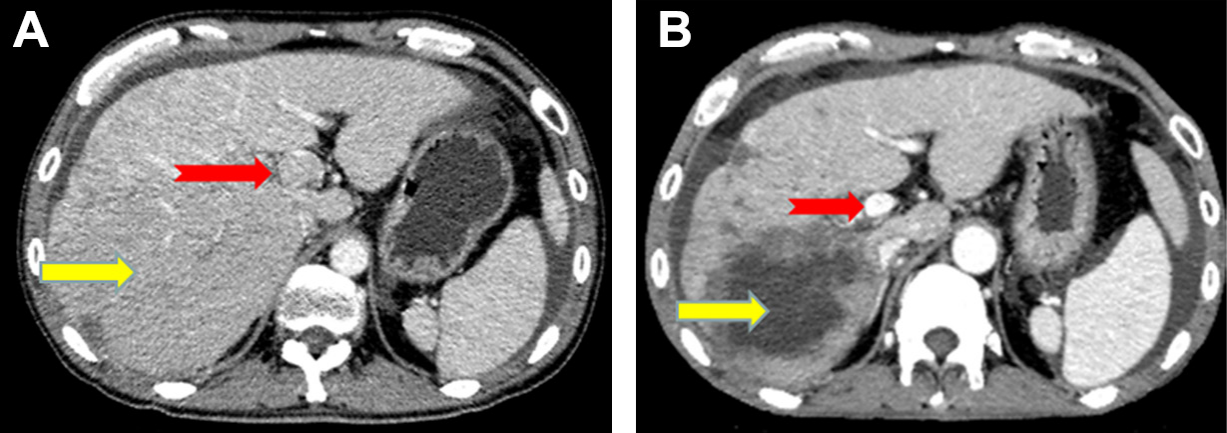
Image of a 48-year-old man with hepatocellular carcinoma and PVTT who showed a partial response after combined apatinib and TACE treatment Contrast-enhanced CT at diagnosis showed a 134 mm diameter hepatocellular carcinoma nodule (yellow arrow) and multiple small metastatic lesions located in the liver, together with PVTT in the left and main portal vein (red arrow). CT images 1 month after diagnosis showed intrahepatic lesions in numerous non-enhanced areas (yellow arrow) and almost complete absence of PVTT without definite enhancement (red arrow).
